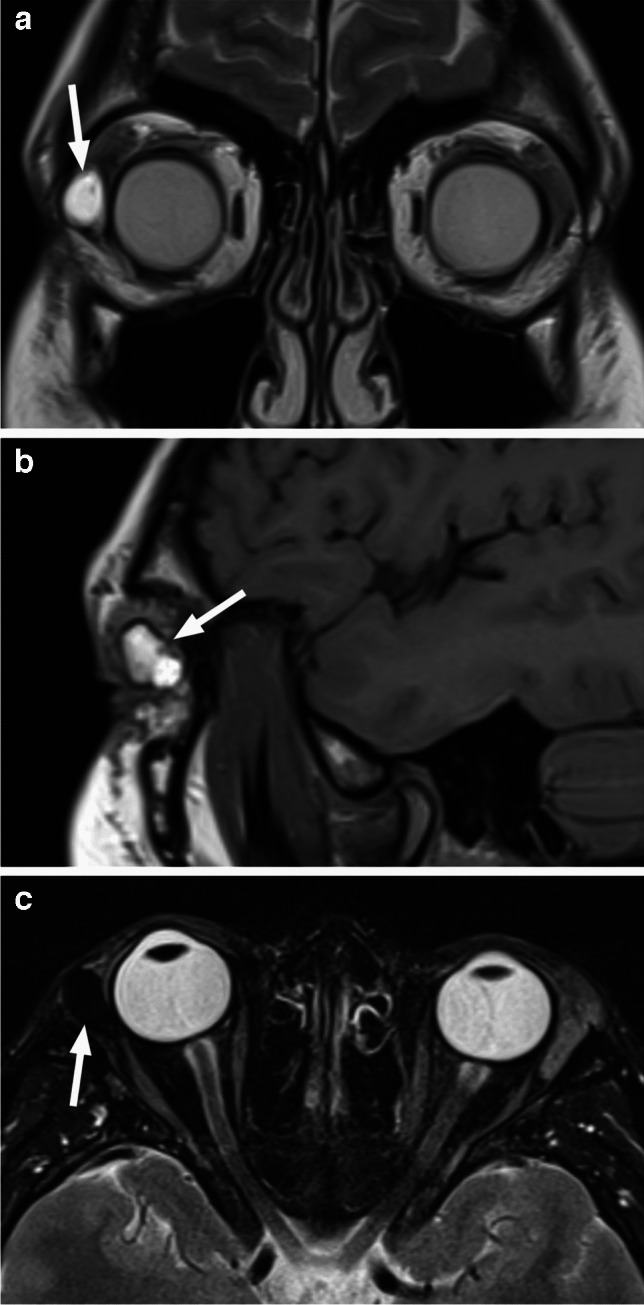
Intraorbital dermoid. Coronal T2-weighted image through the orbits (a) shows an oval extraconal circumscribed mass (arrow) on the right side, adjacent to the lacrimal gland. The lesion exhibits fat-like hyperintensity (arrow) on sagittal T1-weighted image (b) with complete signal suppression (arrow) on fat saturated axial T2-weighted image (c)
